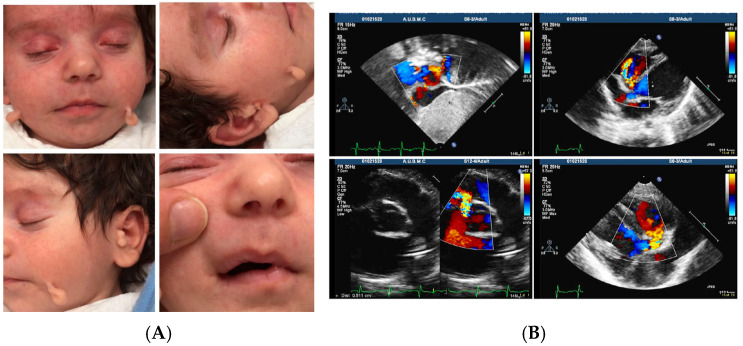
(A): Multiple bilateral accessory tragi and right-sided perioral pits in a 6-month-old girl with Goldenhar syndrome. (B): Echocardiogram with color Doppler imaging of V.6 showing a moderately sized premembranous ventricular septal defect, trace aortic and mitral insufficiencies, and physiological tricuspid and pulmonary insufficiency.
